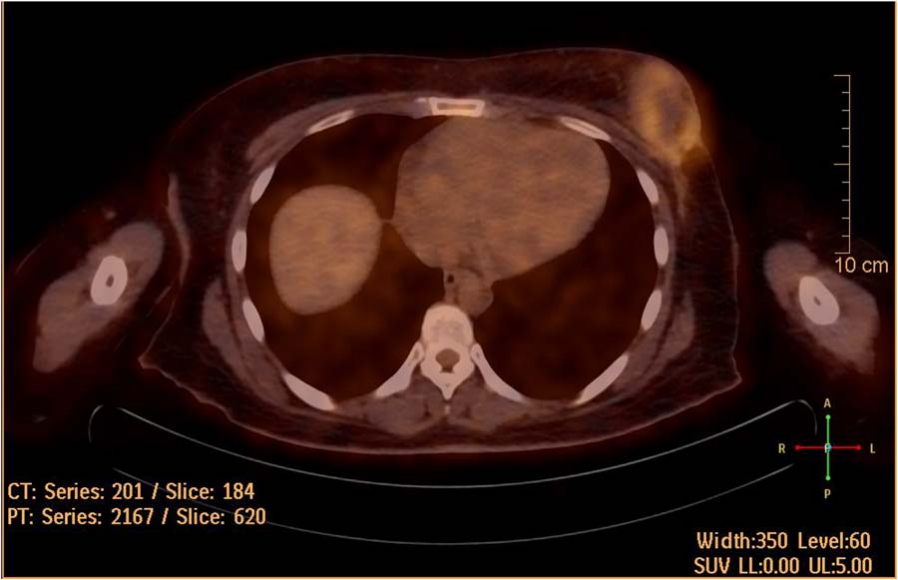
Hypermetabolic left breast mass in last positron emission tomography/computed tomography, following six cycles of IA.
